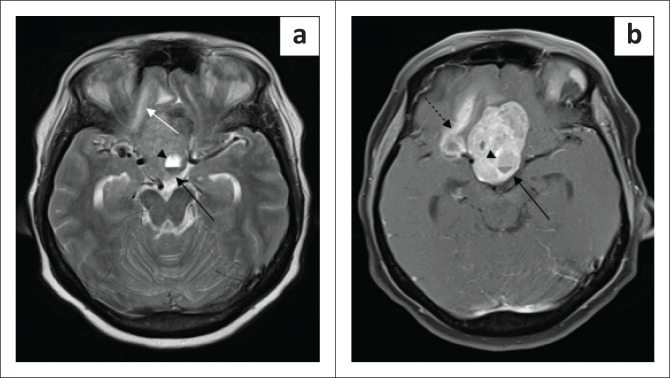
(a, b) Axial T2-weighted and post-contrast T1-weighted MR images showing the heterogeneous sellar-suprasellar mass (black arrows) with T2W hyperintense acute intralesional haemorrhage and fluid levels (arrowheads), and enhancing solid components. Surrounding T2 hyperintensity around the right frontal haematoma (white arrow) is consistent with vasogenic oedema. Peripheral enhancement of the haematoma on post-contrast T1-weighted images (dashed arrow) reflects blood-brain barrier disruption secondary to acute haemorrhage.
